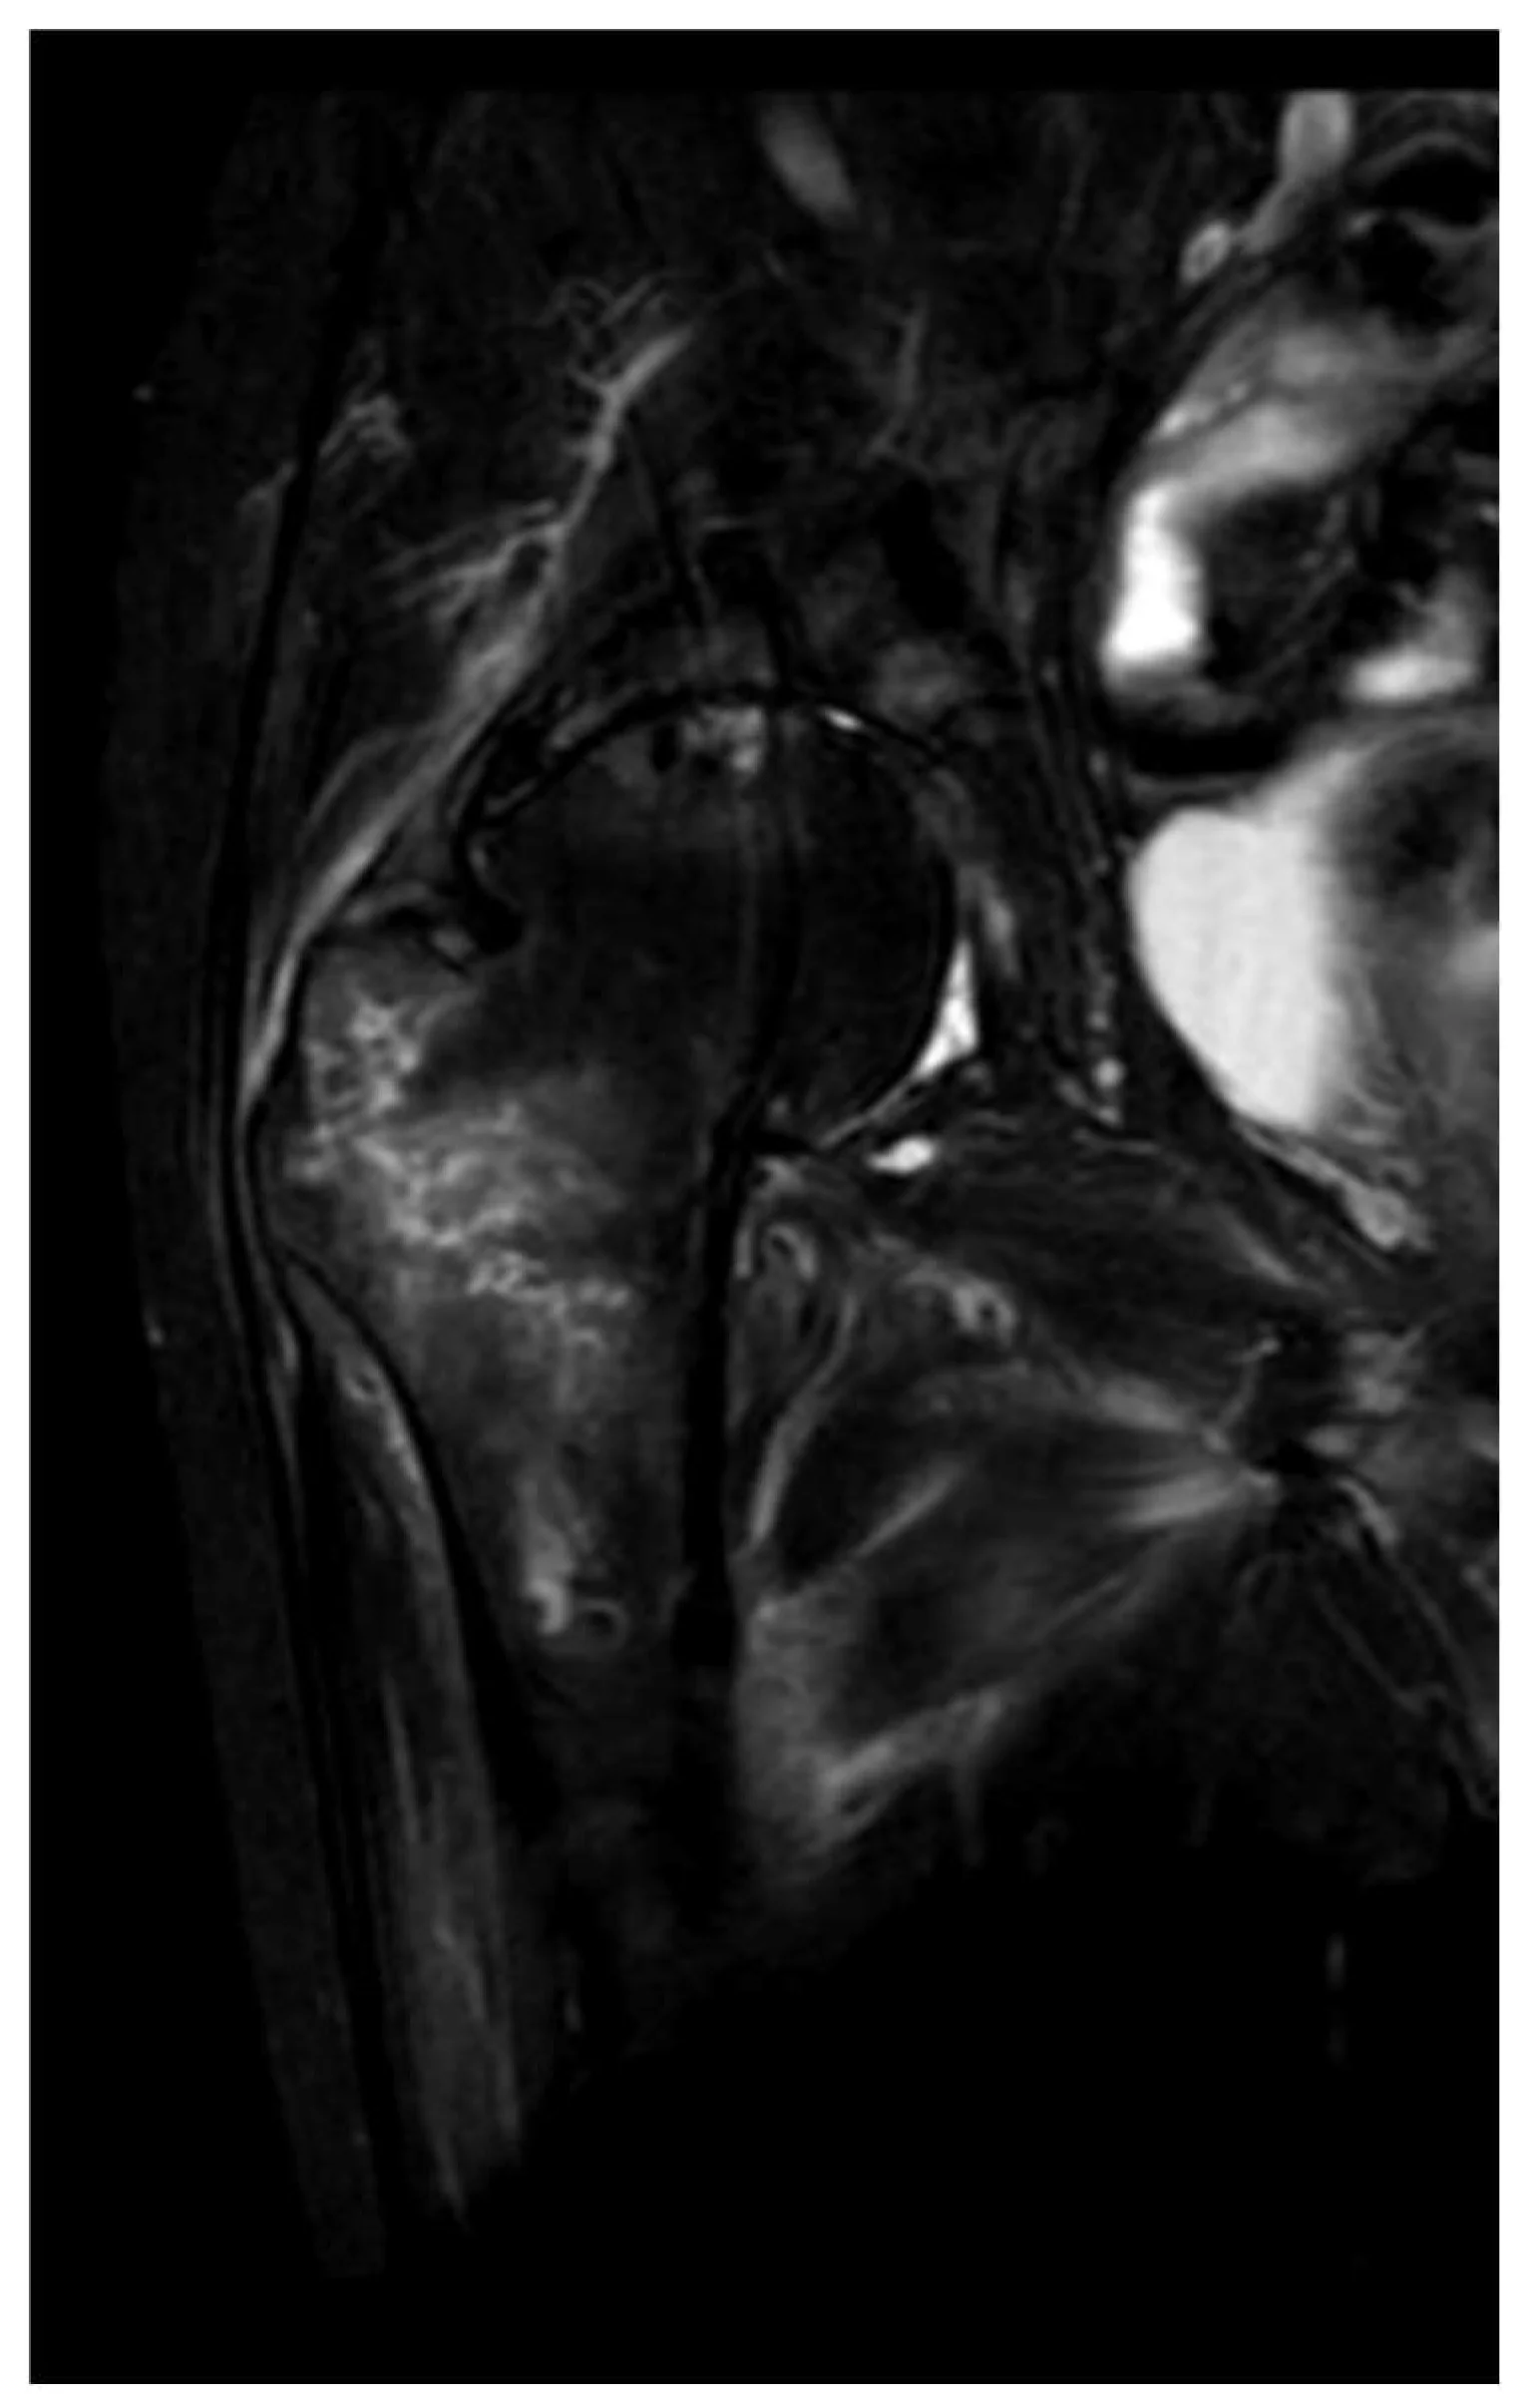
T2-weighted MRI of the right hip in coronal view showing non-displaced fracture of the greater trochanter with marrow edema involving more than 50% of the intertrochanteric region. No obvious cortical breaks of the medial calcar are present.
